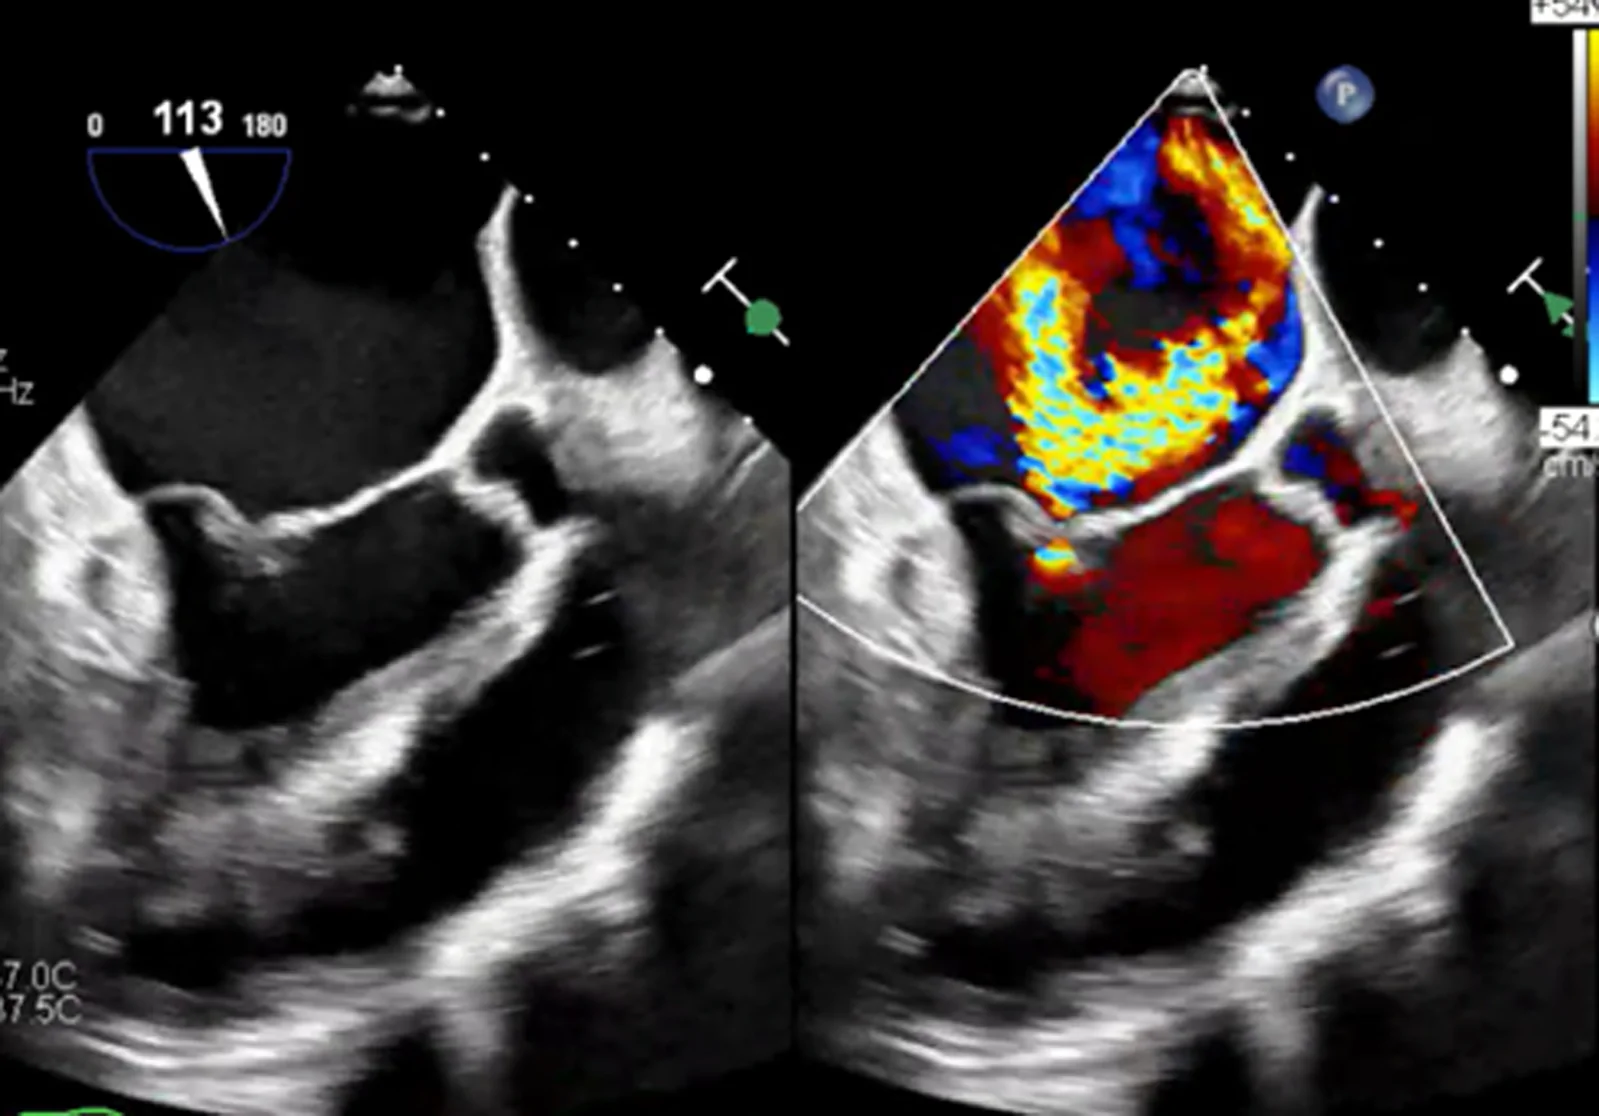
Transesophageal Echocardiography Revealing Severe Mitral Insufficiency With Multiple Eccentric Jets
